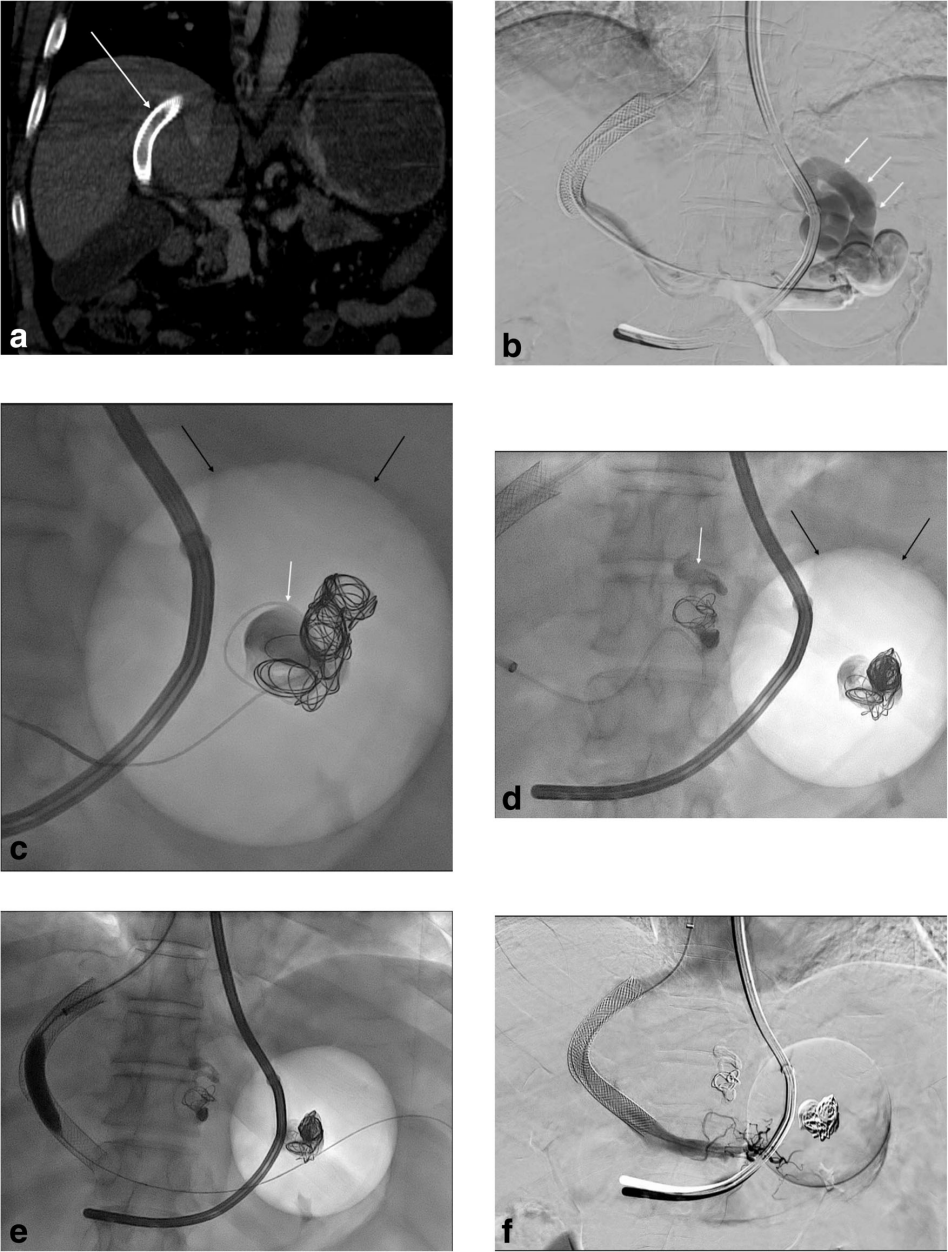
a Contrast-enhanced computed tomography in emergency in a cirrhotic patient with haematemesis shows an occluded transjugular intrahepatic portosystemic shunt (TIPS) (white arrow). b Portography after TIPS recanalisation shows large gastric varices (white arrows). c Embolisation with an ethylene-vinyl alcohol (EVOH) liquid embolic agent (white arrow) and detachable coils of the gastric varices through a coaxial microcatheter. A Blakemore tube with inflated balloon in the stomach is evident (black arrows). d After gastric varices embolisation in the projective correspondence of the Blakemore tube (black arrows), also oesophageal varices were embolised with liquid embolic agent (white arrow) and detachable coils. e After gastroesophageal varices embolisation and an overlapped second stent placement, TIPS recanalisation was completed with high-pressure balloon angioplasty. f Final portography shows a quick opacification of the TIPS and the right atrium as good function of the portosystemic shunt without more evidence of the gastroesophageal varices
